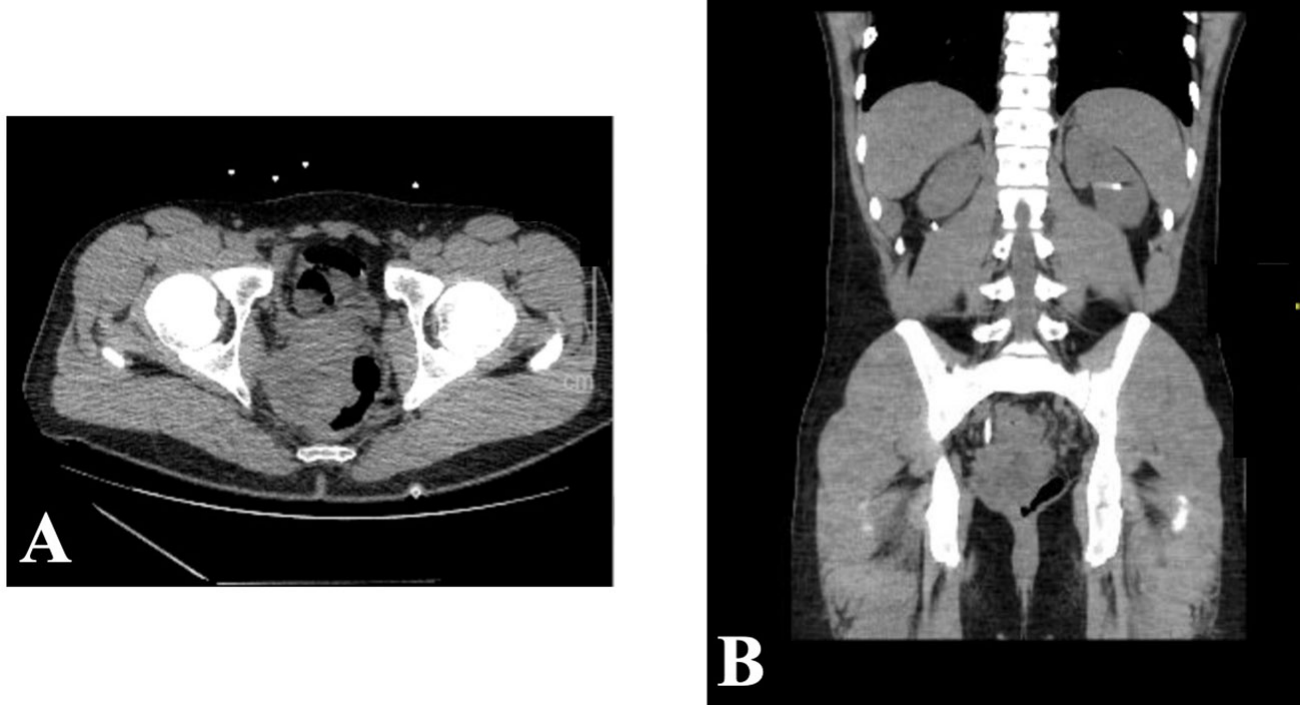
November 2022 CT abdomen and pelvis revealing rectal mass in both axial (A) and sagittal (B) views.
